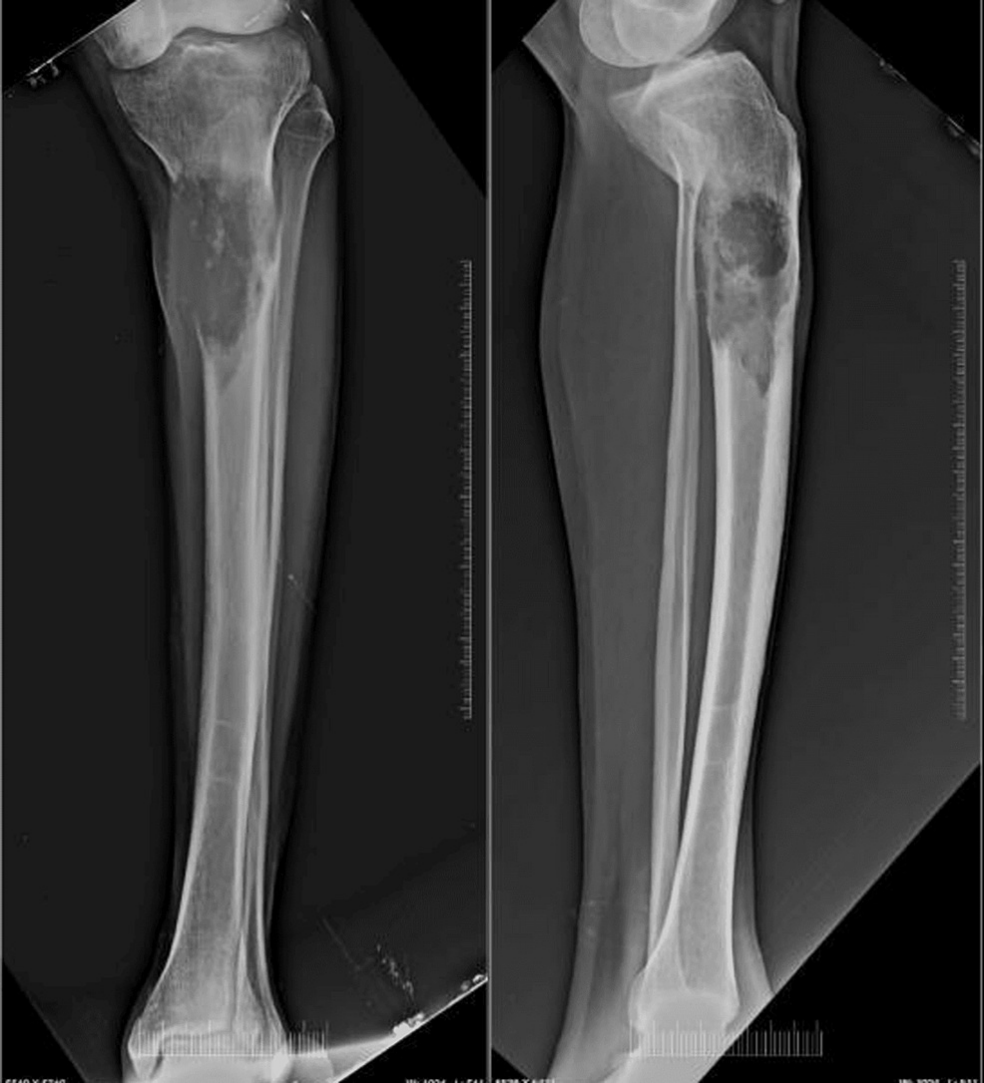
Preoperative radiograph showing lytic lesion in the metaphysio-diaphyseal region A: Anteroposterior view of the leg, B: Lateral view of the leg
